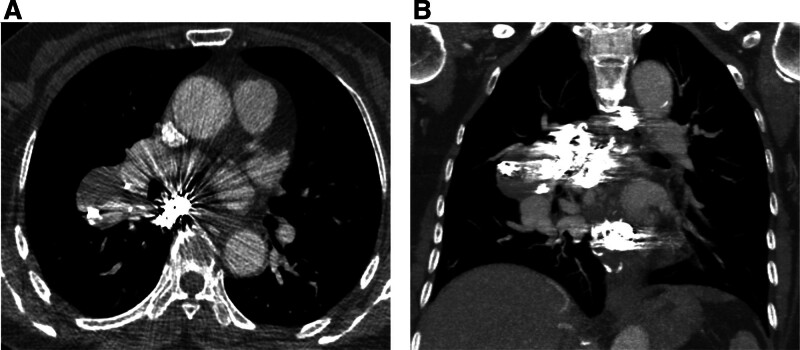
(A) Enhanced CT images demonstrate complete occlusion of the BA, reduction in the size of the largest aneurysm, and formation of an intracavitary thrombus. (B) Coronal CT imaging reveals post-embolization changes in multiple mediastinal arteries, with no evidence of coil migration.
